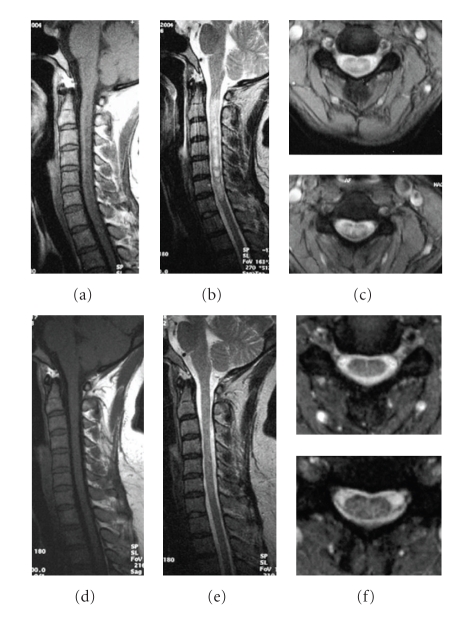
A 40-year-old woman had tetraparesis, C3 sensory level, and urinary incontinence for five months. Pulse methylprednisolone and IVIG were initiated with no resolution. However, ATT greatly improved her condition. The MRI changes are as follows: (a) sagittal T1-weighted MRI showing thickening of the cervical cord, (b) sagittal T2-weighted MRI showing a contiguous area of increased signal intensity spanning C2 to C7, (c) axial T2-weighted MRI showing the strong signal derived from the whole cord, (d) sagittal T1-weighted MRI after 2 years of treatment is normal, (e) sagittal T2-weighted MRI after 1 year of treatment, showing a normal spinal cord, and (f) axial T2-weighted MRI, showing no lesions.
